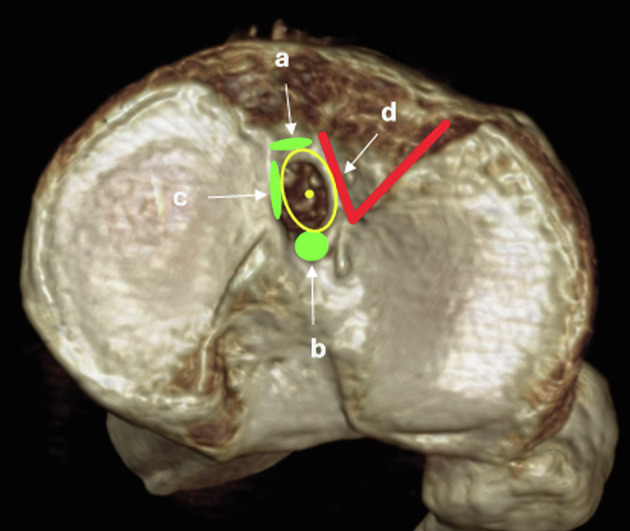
3-D CT scan reconstruction of a right tibial plateau axial view. Bony landmarks for ACL footprint and its center (yellow circle and yellow dot) are represented (a, anterior ridge; b, intertubercular fossae; c, medial intercondylar ridge; d, medial border of the lateral groove).
